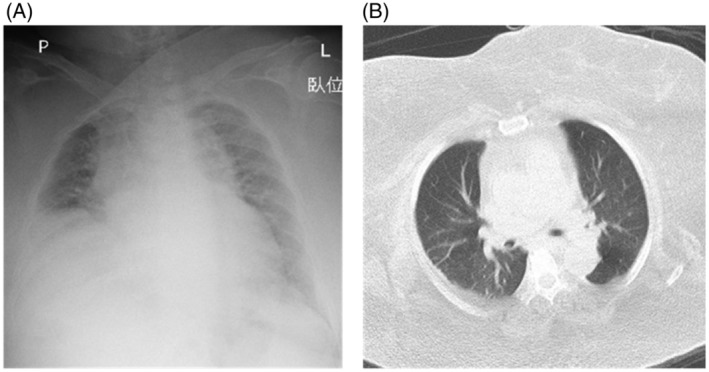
Chest X‐ray and CT of case 3 (A) Chest X‐ray on admission showed an increased cardiothoracic ratio. (B) CT on admission revealed thick subcutaneous fat surrounding the thorax and no obvious infiltration in the pulmonary fields
